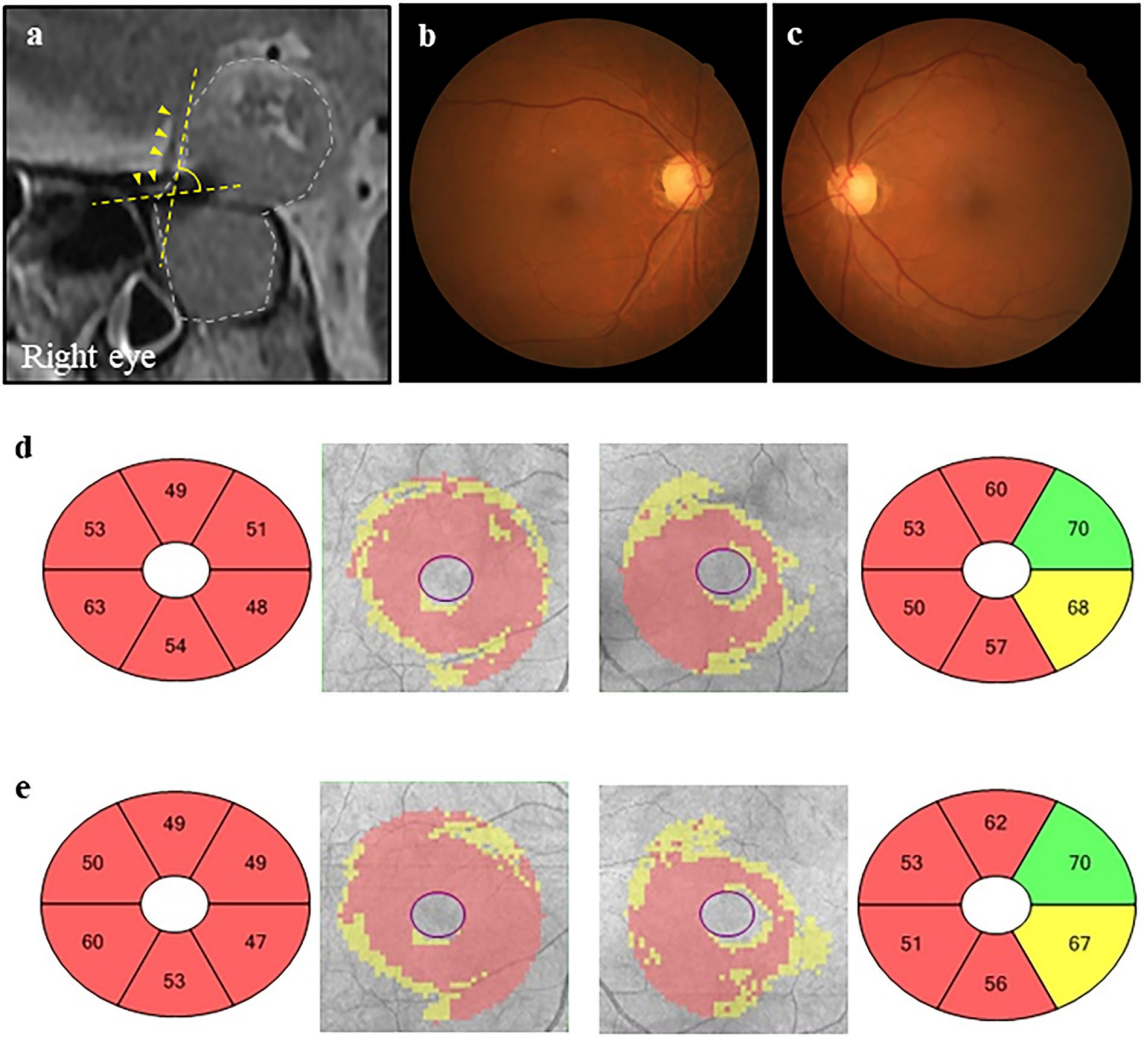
Representative clinical findings of a case with poor visual outcome (case 2). A 74-year-old man with pituitary adenoma with optic nerve bending in the right eye. The preoperative and postoperative best-corrected visual acuities (BCVAs) were 0.82 and 0.10 in the right eye and 0.70 and − 0.08 in the left eye logarithm of the minimum angle of resolution units, respectively. (a) Preoperative sagittal T2-weighted magnetic resonance images. The angle indicated by yellow dotted lines is the optic nerve–canal bending angle (ONCBA); yellow arrowheads indicate optic nerve, and white dotted lines indicate a tumor. The ONCBAs of this case were 73° in the right eye and 39° in the left eye. Therefore, the right eye is the one with optic nerve bending. (b, c) Color fundus photograph showed normal appearance at preoperative visit (B, right eye; C, left eye). (d, e) Ganglion cell layer (GCL) + inner plexiform layer (IPL) deviation map and sector graph of the right eye (left 2 panels) and left eye (right 2 panels) (d, preoperative; e, postoperative visit). A decrease in preoperative GCL + IPL thickness of the right eye is observed in all sectors. A decrease in preoperative GCL + IPL thickness of the left eye is observed in the predominantly nasal region. Postoperative GCL + IPL thickness in almost all sectors was more unremarkable than preoperative GCL + IPL thickness in both eyes.
